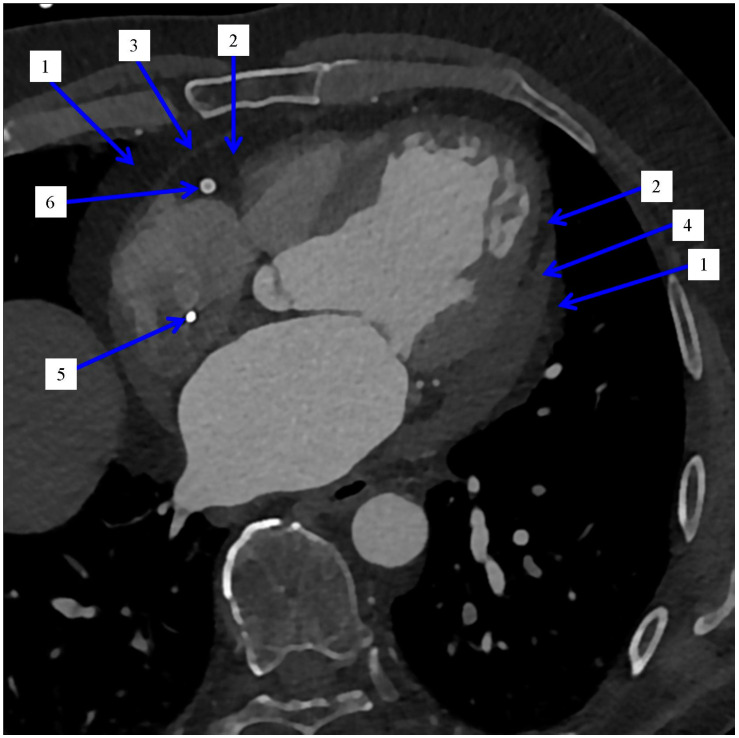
Cardiac computed tomography in a patient after interventional treatment. Multiplanar oblique reconstruction. Blue arrows indicate: 1. Pericardial adipose tissue. 2. Epicardial adipose tissue. 3. Pericardium. 4. Fluid in the pericardium. 5. Cardiac pacemaker electrode in the right atrium. 6. Right coronary artery stent.
